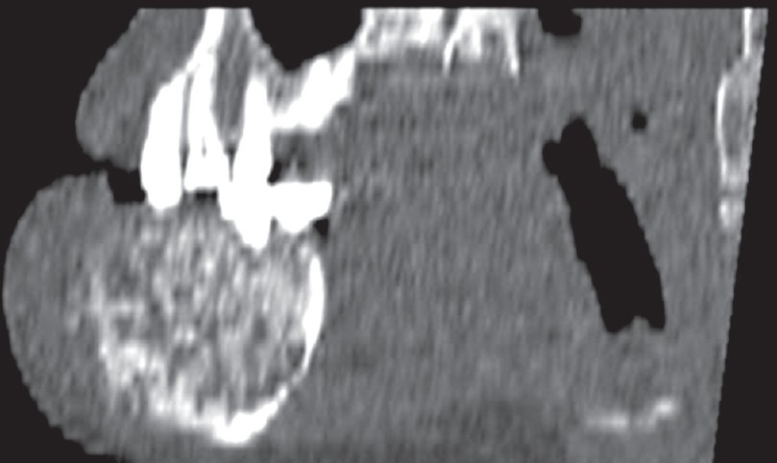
Sagittal MPR CT image of the mandible with bone window settings shows an expansile, solid, mixed radiodense-radioopaque lesion with poorly defined margins in the anterior mandible, with destruction of the cortices and extension into the soft tissues
